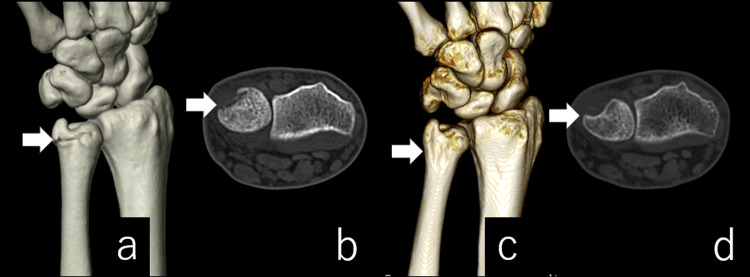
Computed tomography scan. (a) and (b) are CT images two weeks after the injury; (c) and (d) are postoperative images. The step-off at the fracture site was shaved and a groove was created.
